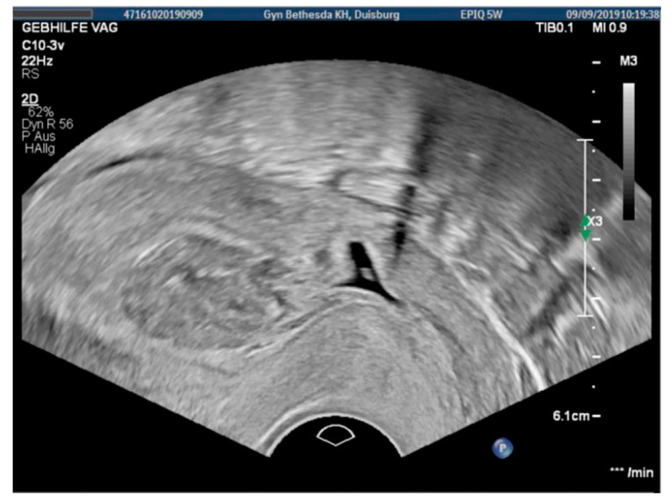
Transvaginal ultrasound showing a niche pregnancy with 6 weeks of gestation.
